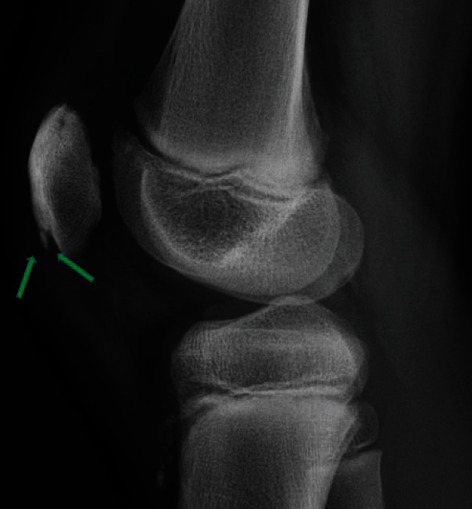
X-ray 1. Lateral view of the right knee. Green arrows shows the Sinding-Larsen and Johansson disease, initial radiological findings.
